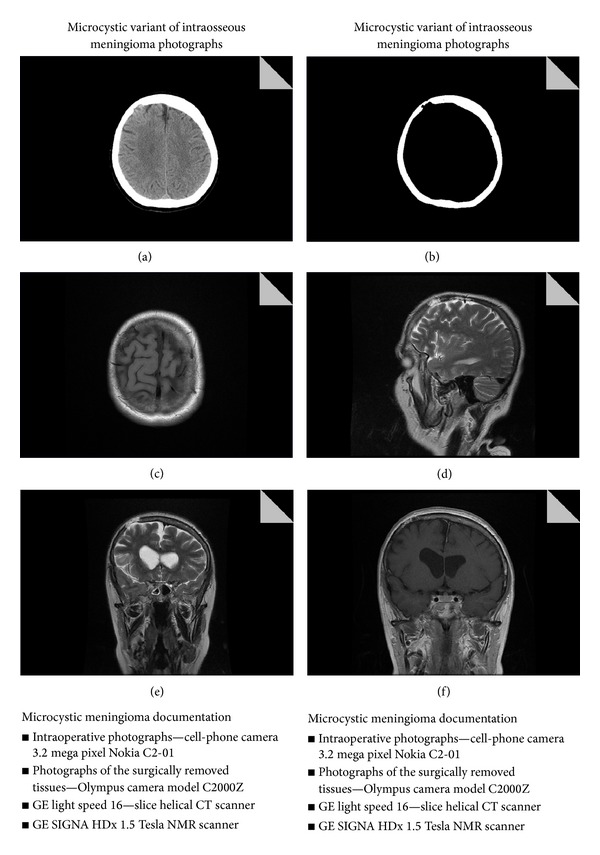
Imaging characteristics: (a) head CT scan, axial projection, and osteolytic defect in the right frontal area in the location of the bone tumour; (b) head CT scan, axial projection, bone window, and osteolytic defect in the right frontal area; (c) MR of the brain, axial projection, T1 SE, and the area of mixed signal intensity inside the bone in the right frontal area; (d) MR of the brain, image in the sagittal plane, and T2 FSE hyperintense area in the right frontal area; (e) MR of the brain, image in the coronal plane, and T2 FSE, hyperintense area in the right frontal area; (f) MR of the brain, image in the coronal projection, T1 following the application of contrast agents, and contrast enhancement features involving only the bone structure.
